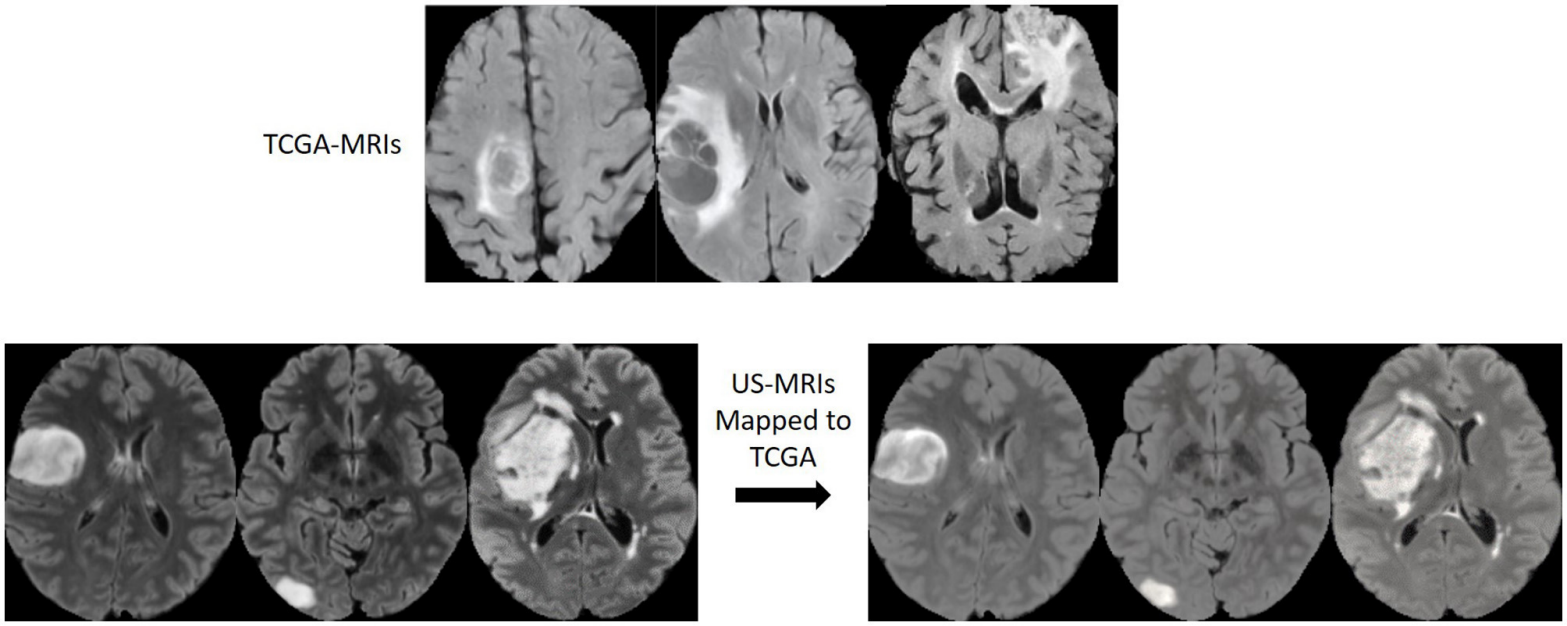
MR image slices before and after the domain mapping. (Top) Original FLAIR MR images from TCGA dataset. (Bottom left) Original FLAIR MR images from US dataset. (Bottom right) Corresponding FLAIR MR images after domain mapping.
